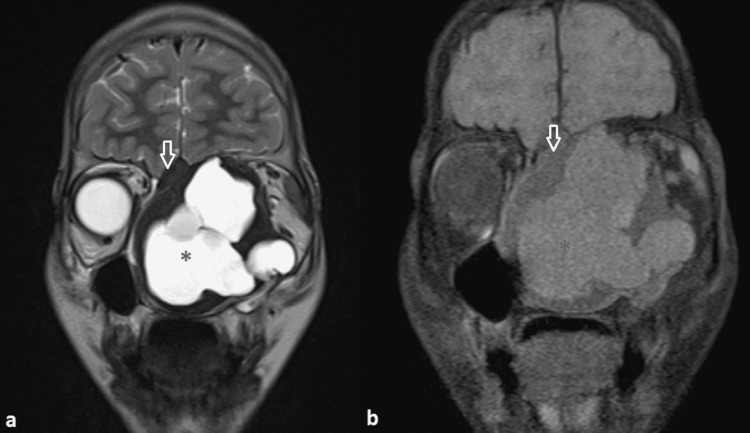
Coronal MRI images (a) T2 weighted image and (b) T1 weighted MRI image A lytic expansile lesion is seen involving the bilateral nasal cavity with a central cystic T1/T2 hyperintense (black asterisk) component and peripheral T1/T2 hypointense regions (white open arrow). MRI - Magnetic Resonance Imaging
